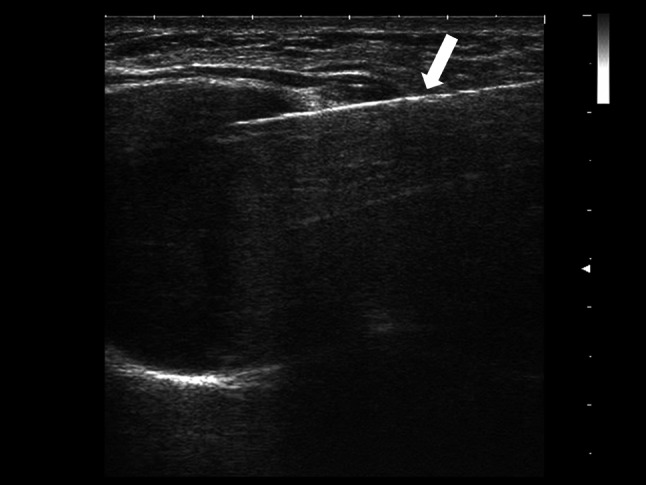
Female patient of 38 years old. B-mode scan during ultrasound guided core-needle biopsy (arrow). Chest wall sarcoma appears as a homogeneous, hypoechoic mass with irregular and infiltrative margins
